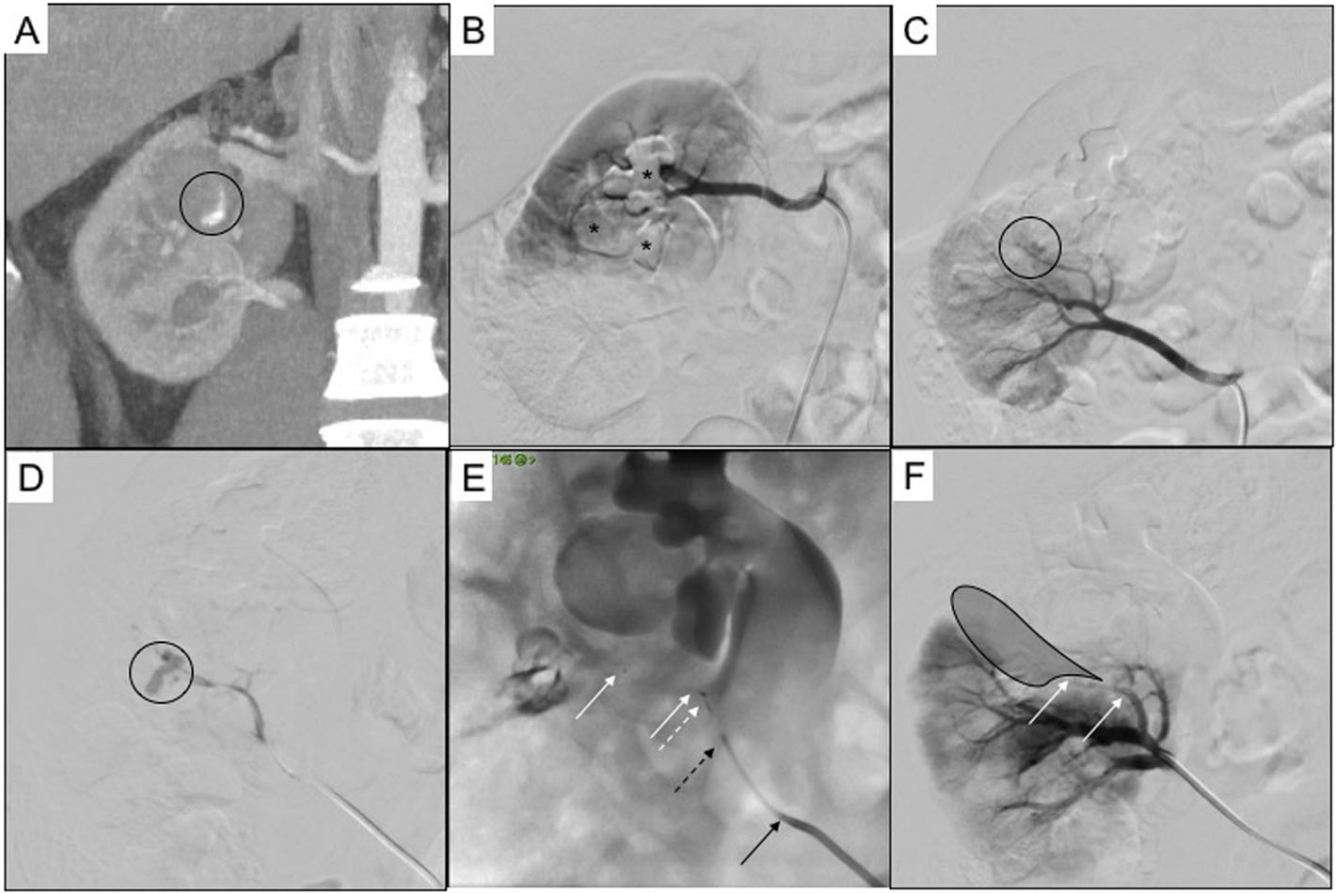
Patient 1. 24 years old man with double renal artery and urinary stones causing right pielectasia, treated with nephrostomy. a arterial phase CT scan reconstruction in coronal plane showing intracaliceal active bleeding (black circle); b DSA performed from the cranial renal artery showing no angiographic bleeding signs, previous CT contrast agent mixed with clots is appreciable in the calixs (black asterisks); c DSA performed from the caudal renal artery showing extraluminal blush (black asterisk) from the interlobar branch of the inferoanterior segmental artery; d superselective lobar microcatheterization confirming bleeding (black asterisk); e superselective embolization with MVP 3Q (white arrows indicate distal and proximal radiopaque markers of the MVP, white dotted arrow indicates the pusher wire radiopaque extremity after detachment, black dotted arrow indicates the 2.7Fr Progreat microcatheter, black arrow indicates the 5Fr diagnostic Cobra 1 catheter); f final DSA control showing no more angiographic bleeding (black shadow indicates the ischemic area, white arrows indicate distal and proximal radiopaque markers of the MVP)
